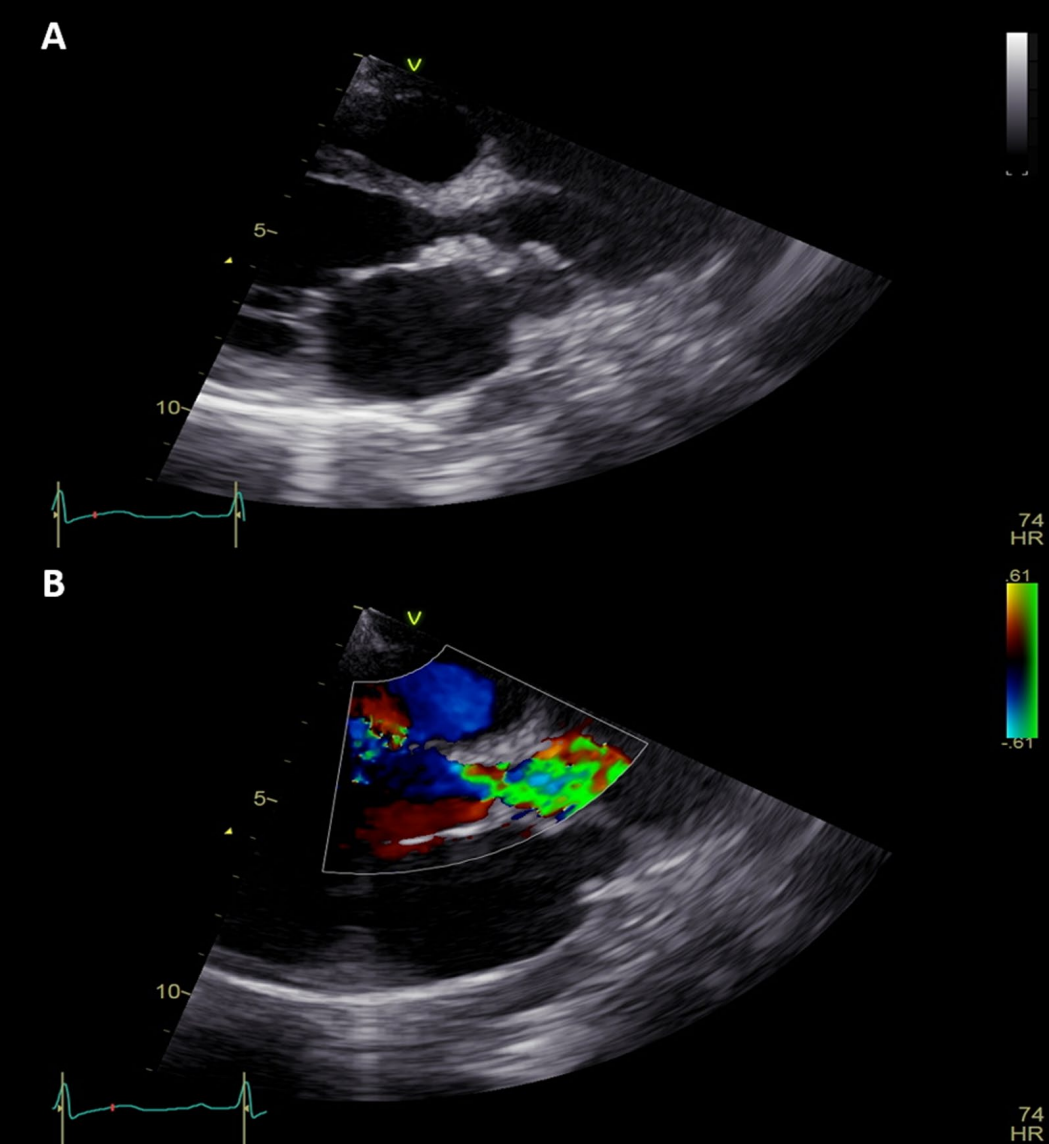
a Parasternal long axis view showing narrowed aortic root on 2D, and b turbulence on color Doppler
